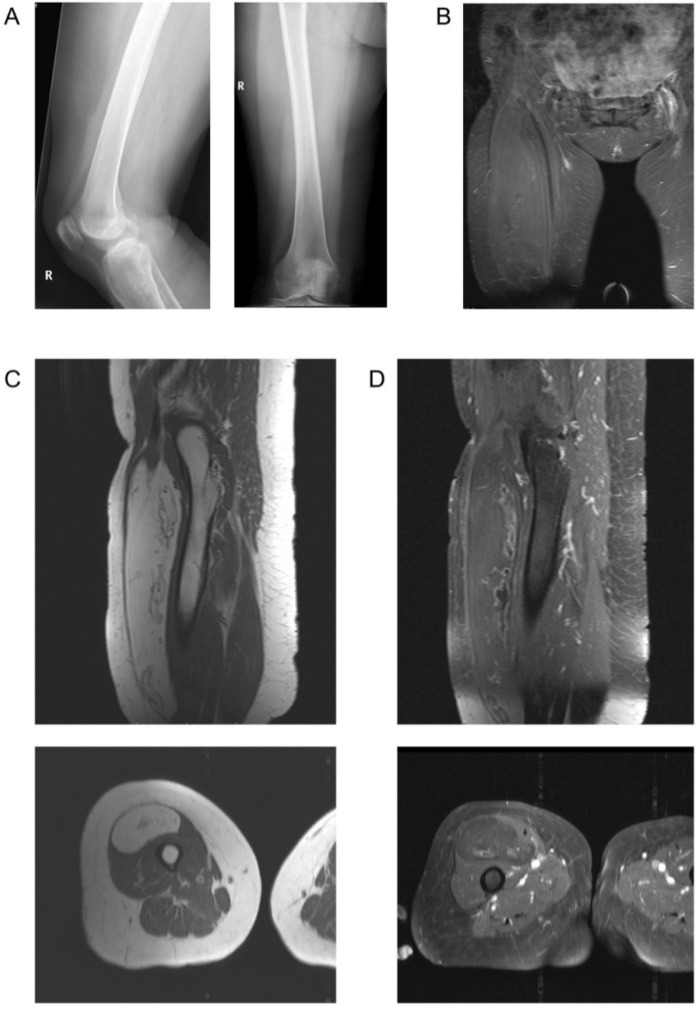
Images of a patient with low-grade liposarcoma (atypical lipomatous tumor) and an initial report describing lipoma. (A) X-Ray in lateral and a.p. view. (B) Coronal TIRM (STIR) sequence. (C) T1-weighted image precontrast in sagittal and axial plane. (D) T1-weighted image postcontrast with fat saturation.
